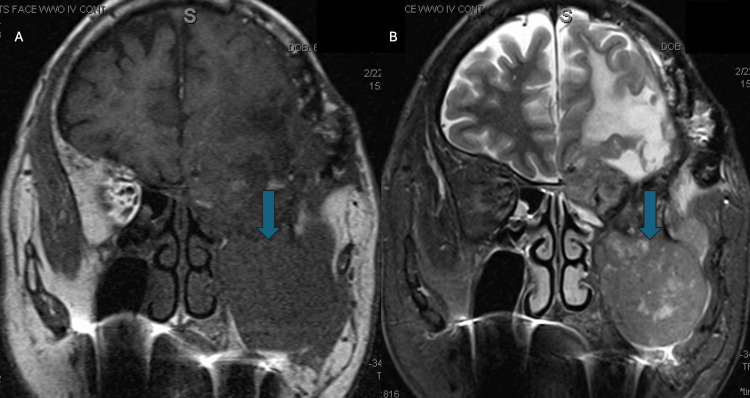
Skull-base anaplastic meningioma. (A) Coronal post-contrast T1-weighted MRI demonstrating a large, heterogeneously enhancing left spheno-orbital mass (blue arrow) with extension into the orbit, skull base, and adjacent soft tissues (blue arrow). The tumor shows irregular margins and avid enhancement, consistent with high-grade meningioma.
